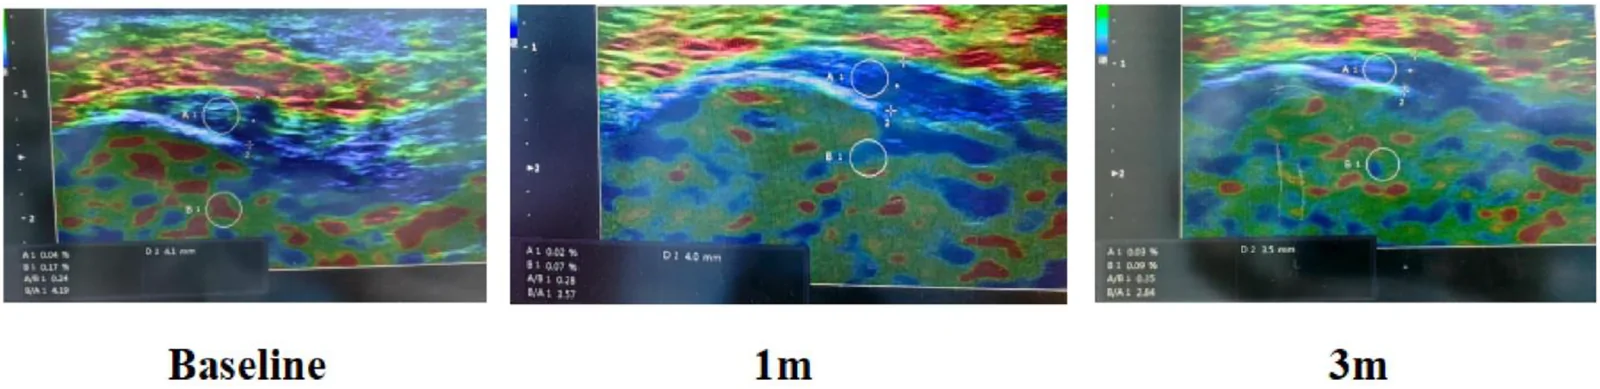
Representative ultrasonographic measurements of plantar fascia strain ratio and thickness.
